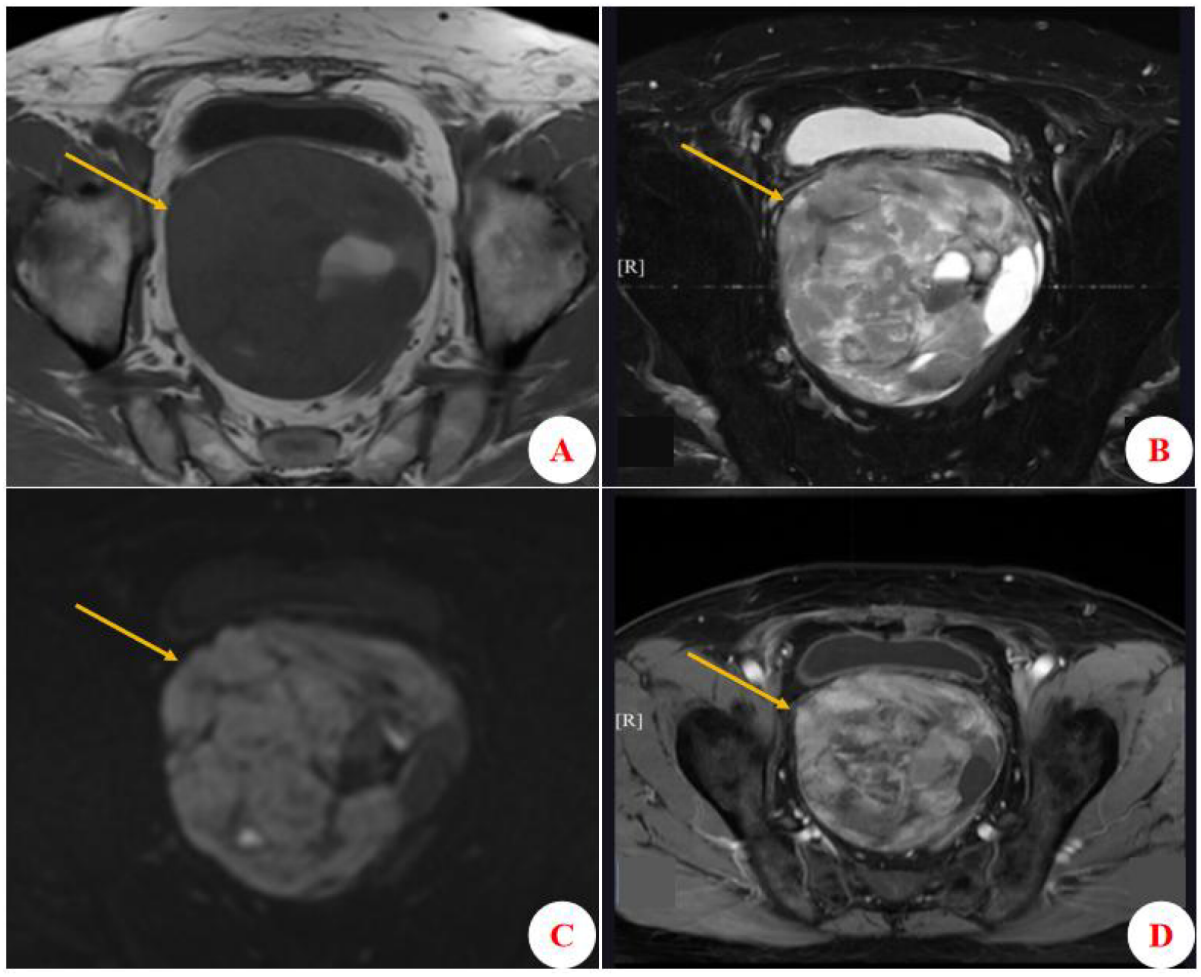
Pelvic MRI at recurrence (7 years postoperative). (A) Axial T1-weighted imaging (T1WI) shows a large pelvic mass (approximately 14.80 × 10.20 × 9.40 cm), with hypointense signal. (B) Axial T2-weighted imaging (T2WI) demonstrates a large heterogeneous signal mass. The lesion is predominantly solid with multiple cystic areas. (C) Diffusion-weighted imaging (DWI) reveals diffusional restriction within the mass. (D) Axial contrast-enhanced T2WI reveals marked compression of the bladder and the rectosigmoid junction by the enhancing mass.
